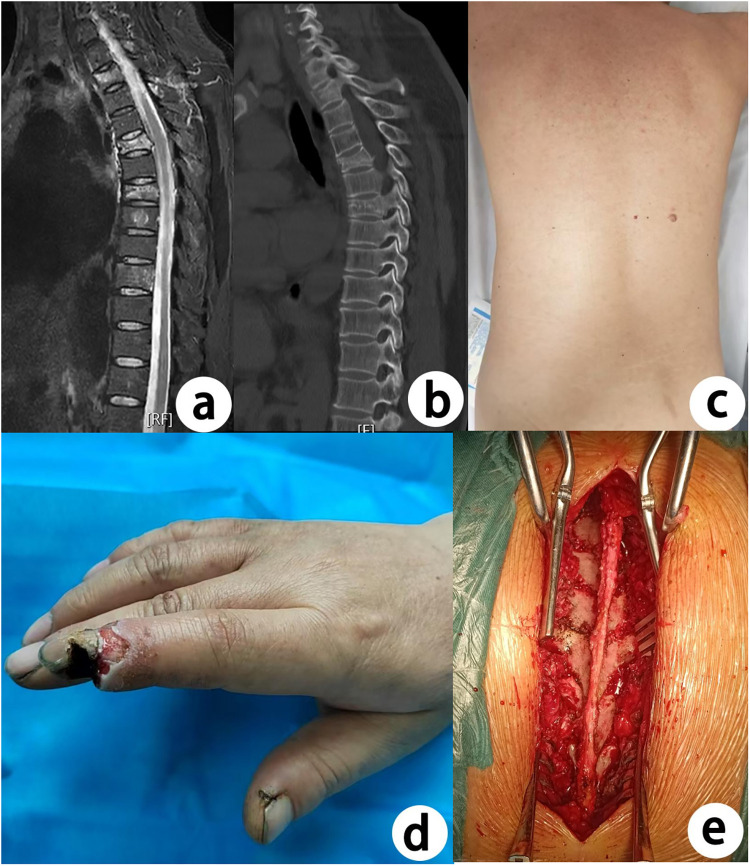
(a) preoperative MRI examination revealed high signal intensity in the T1, T4, and T6 vertebrae; (b) preoperative CT examination demonstrated fractures of the T4 and T6 vertebrae, along with a collapse of the posterior wall of the vertebrae; (c) preoperative appearance of the patient's back: skin intact, no direct traumatic factors; (d) electric burns injury on the patient's left hand; (e) intraoperative appearance (T3-T7).
